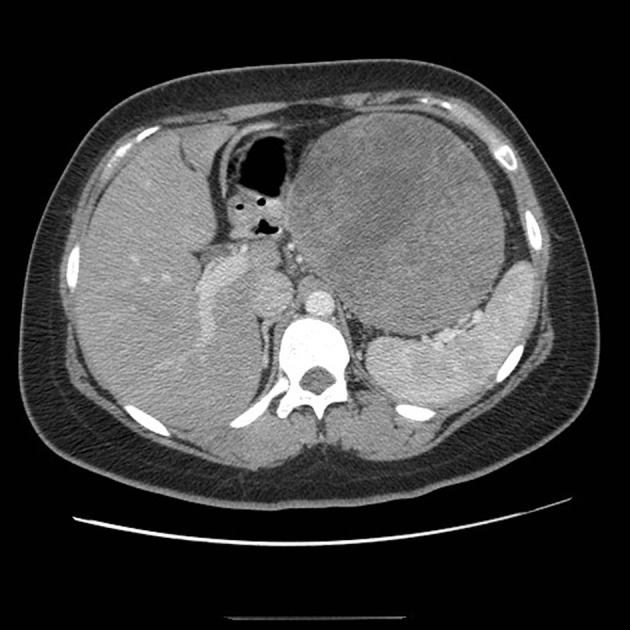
CT scan of the abdomen demonstrating a large mass arising from the tail of the pancreas.
